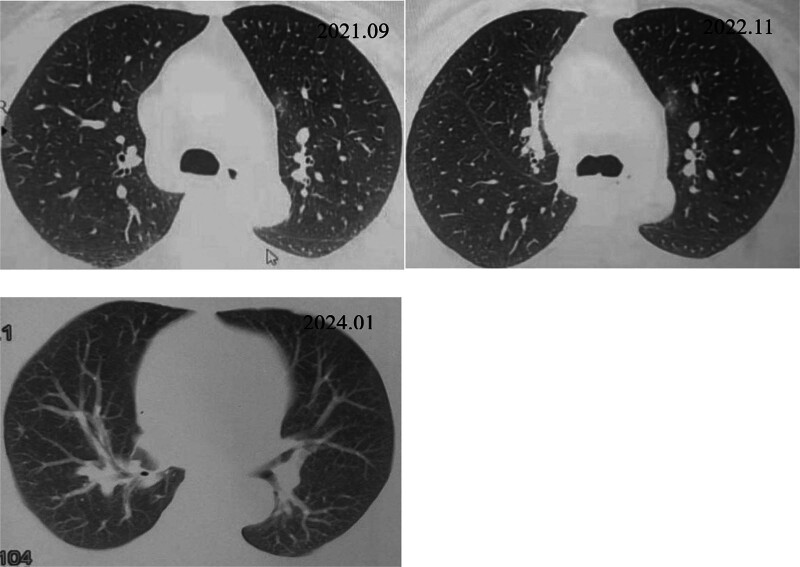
The CT images show changes in lungs before and after treatment.
